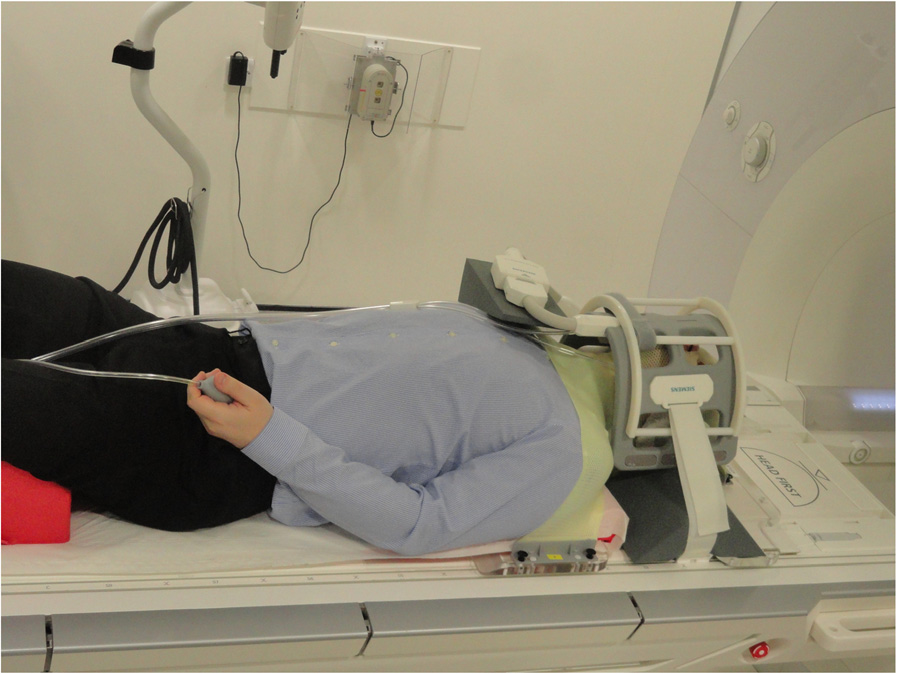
INSIGHT study MRI patient set up (photograph shows one of the study authors during study design and testing). Study patients are positioned on a flat top MRI couch using the same baseplate, neck rest, shoulder rest, and thermoplastic mask as used for radiotherapy treatment planning and delivery. A single multi-channel phased array flexible coil placed over the thermoplastic mask is used to provide anterior coverage of the anatomy of interest, whilst spine-array coils built-in to the couch provide posterior coverage. This set up allows for rapid patient positioning and MRI data acquisition in the radiotherapy treatment position which facilitates subsequent image co-registration with longitudinal MRI scans as well as with CT and PET/CT data
